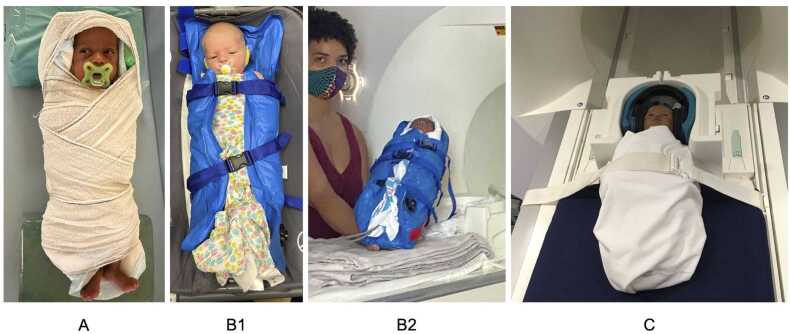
Immobilization approaches common for infant scanning. A. Swaddling the infant in an MRI-safe wrap or blanket; B. A vacuum immobilizer on an infant up close (B1) and on the scanner bed with leads attached for external monitoring (B2). C. A strap that prevents awake or older infants from rolling off the table (swaddle is optional).
